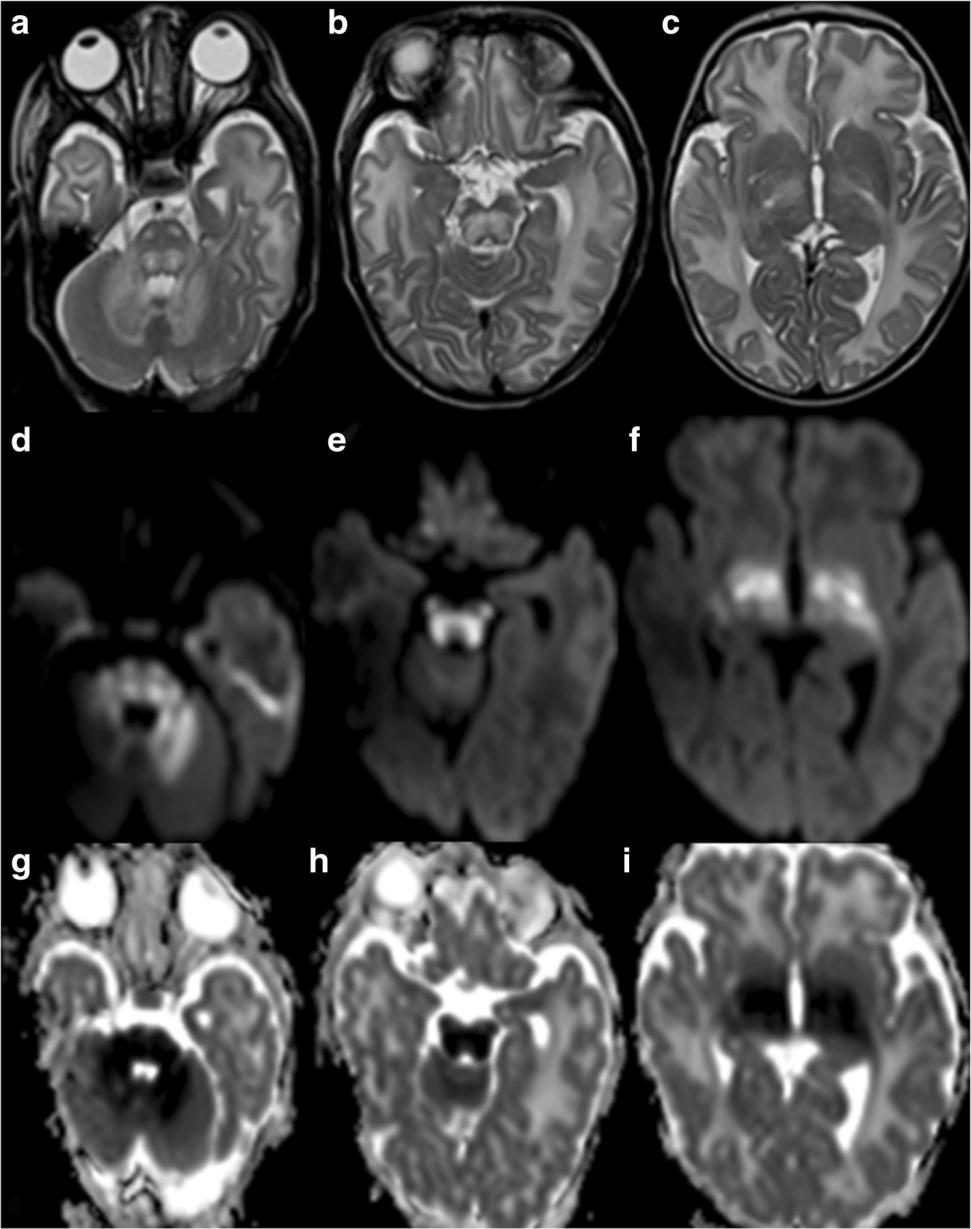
a-c, Axial T2-weighted MR images of a 20-day-old male term neonate with maple syrup urine disease show swelling and hyperintense signal of the cerebellar white matter, dorsal pons, corticospinal tracts along its course in the basis pontis, midbrain, and posterior limbs of the internal capsule, and thalami. d-f, Trace of diffusion images and g-i, Apparent diffusion coefficient (ADC) maps of the same newborn reveal bright DWI-signal and matching low ADC values, respectively, in the cerebellar white matter, dorsal pons, corticospinal tracts in the basis pontis, midbrain, and posterior limb of the internal capsule, and thalami representing restricted diffusion/cytotoxic edema compatible with extensive ongoing injury to the myelinated parts of the brain
